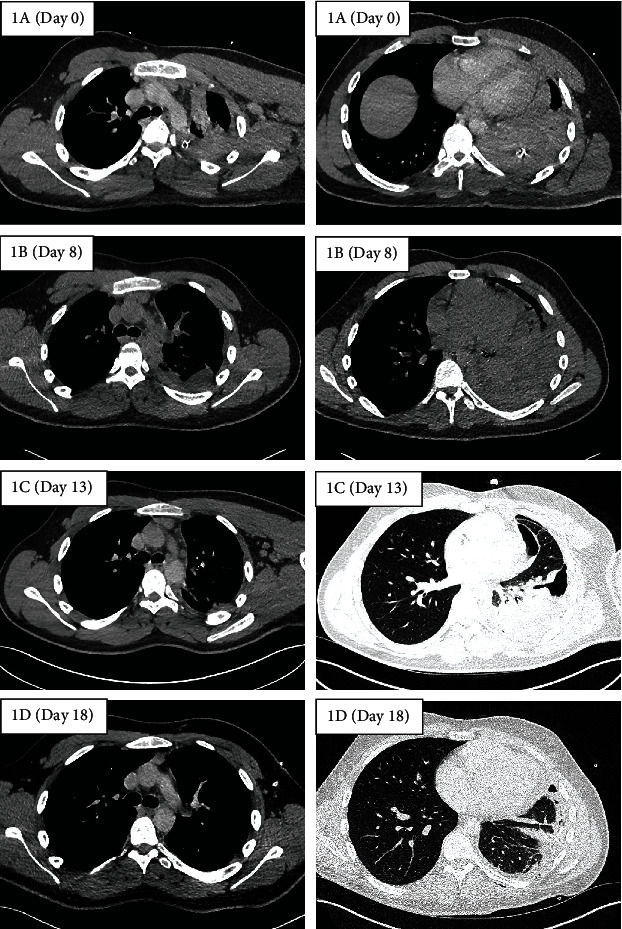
Serial axial chest computed tomography (CT) demonstrating reduction in size of the left sided haemothorax over time. Images on the left and right represent CT slices taken at the level of the carina and the lung base, respectively. Day 0 refers to day of admission.
